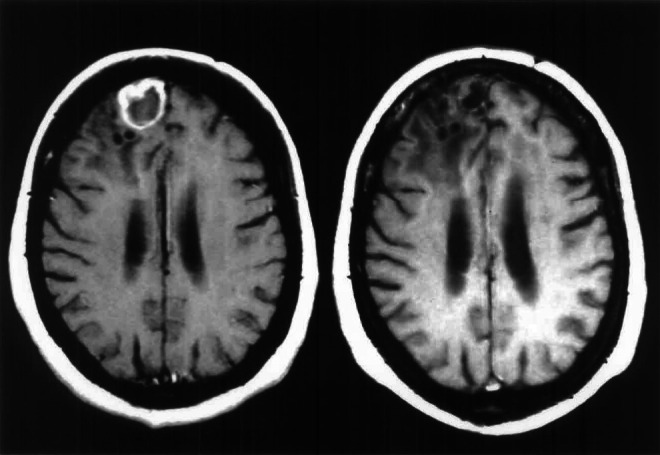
Glioma grade II patient transforming to GBM. This MRI shows a patient with grade II glioma, which had transformed to GBM. This is an ongoing response to TMZ and PCB at dose level 3 at 14+ months.
